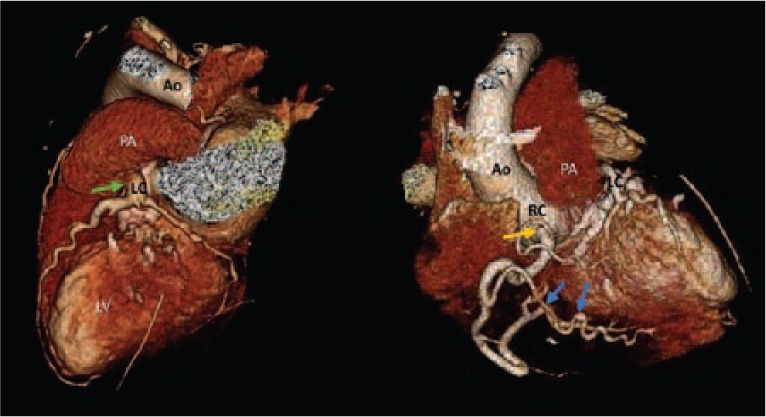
Three-dimensional rendering of coronary CT angiogram demonstrating origin of the right coronary artery (RC) which originates in the aorta (Ao) and provides collaterals across the left ventricle (LV) to supply the left coronary artery (LC) that originates in the pulmonary artery (PA).
